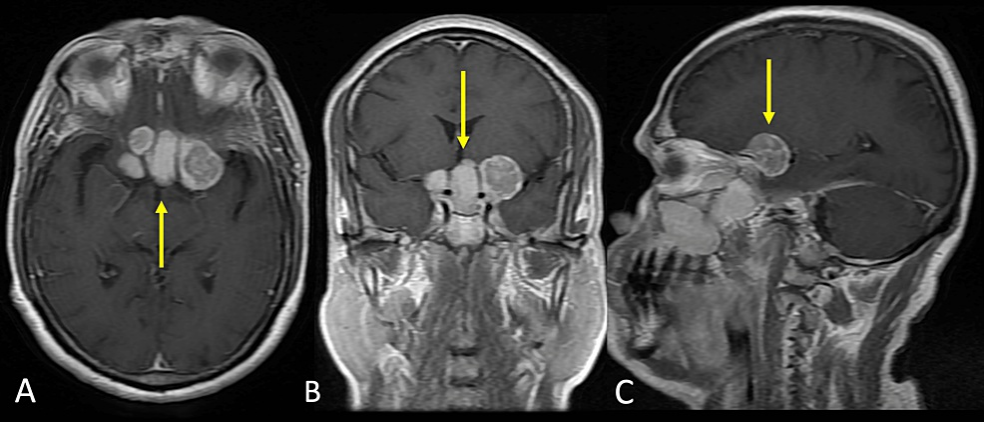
Magnetic resonance imaging with contrast images A. Axial T1WI contrast section showing multiple heterogeneously enhancing mass in the supra and parasellar region suggesting intracranial involvement of disease (yellow arrow). B. Coronal T1WI contrast section showing multiple heterogeneously enhancing mass in the supra and parasellar region suggesting intracranial involvement of disease (yellow arrow). C. Sagittal T1WI contrast section showing heterogeneously enhancing mass in the suprasellar region suggesting intracranial involvement of disease (yellow arrow).
